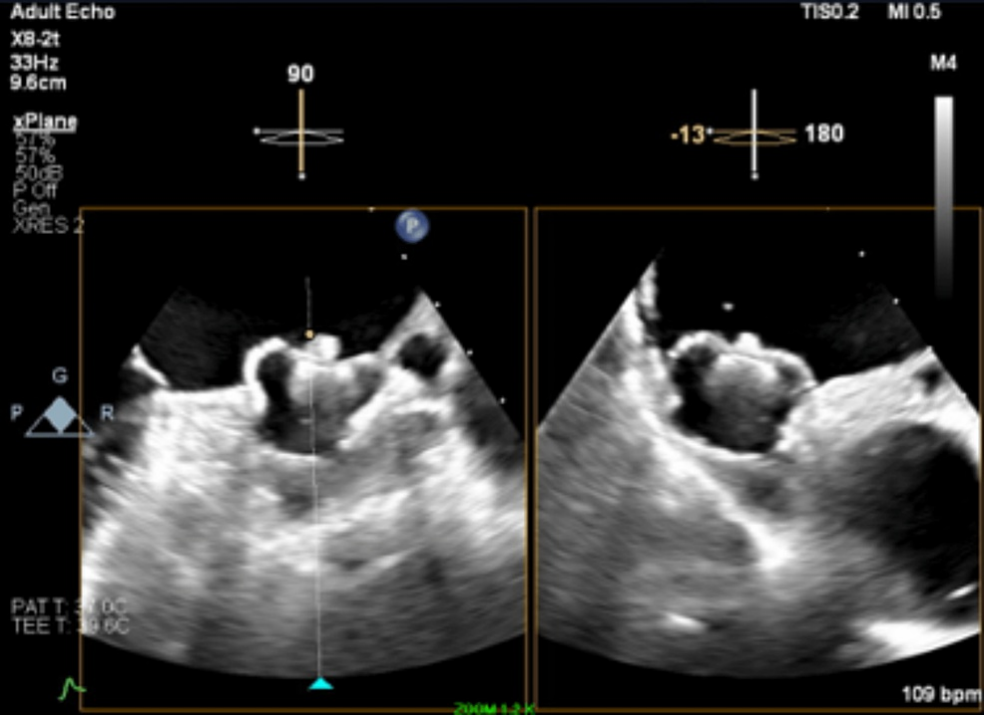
Transesophageal echocardiogram showing final stable position of the Watchman FLX device in the left atrial appendage
